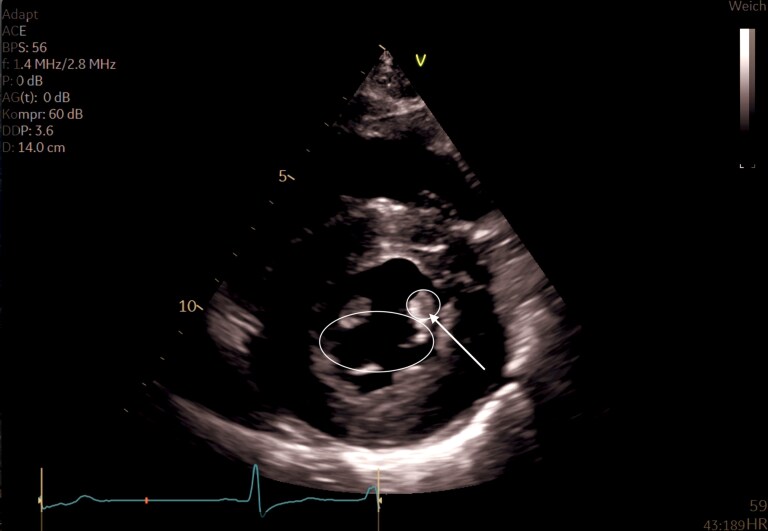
Parasternal short-axis view demonstrating the ‘lip-nevus sign.’ The mitral valve leaflets appear as opposing ‘lips,’ while a markedly hypertrophied anterolateral papillary muscle (arrow) is already visible at the level of the mitral valve, resembling a central ‘nevus.’ This finding strongly suggests anomalous papillary muscle insertion.
